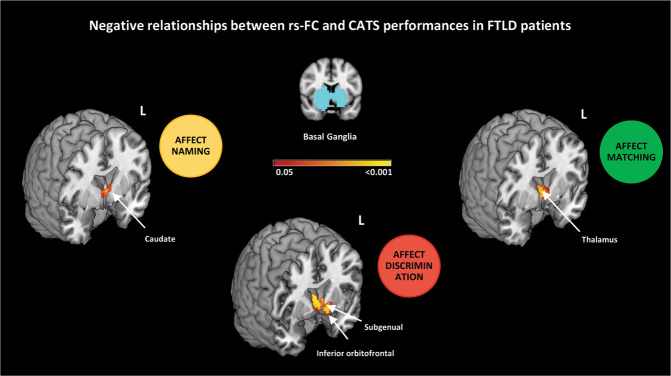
Independent component analysis. Negative relationship between resting state functional connectivity and CATS scores in FTLD patients. Negative relationships are shown in warm colors. Results are overlaid on the Montreal Neurological Institute (MNI) standard brain and displayed at *p* < 0.05 Family-wise error corrected for multiple comparisons. Age at MRI, sex and education were included in the model as nuisance variables. rs-FC resting state functional connectivity, L Left. Color bar represents *p* values.
